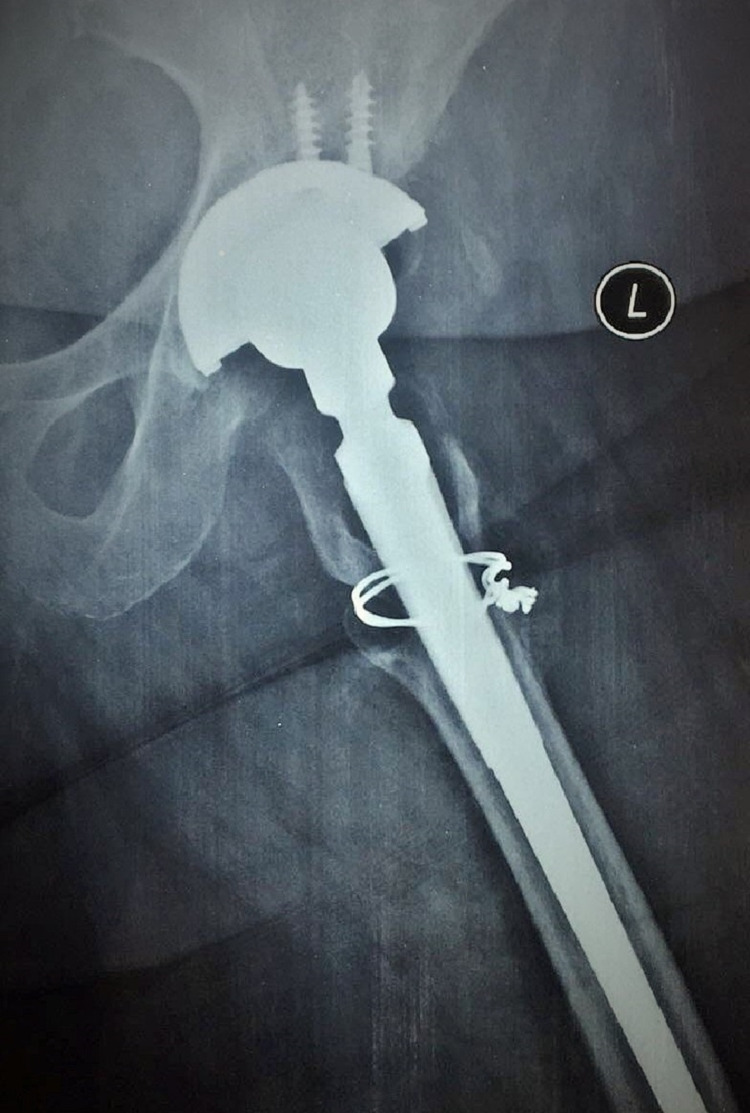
Preoperative radiographic evaluation
